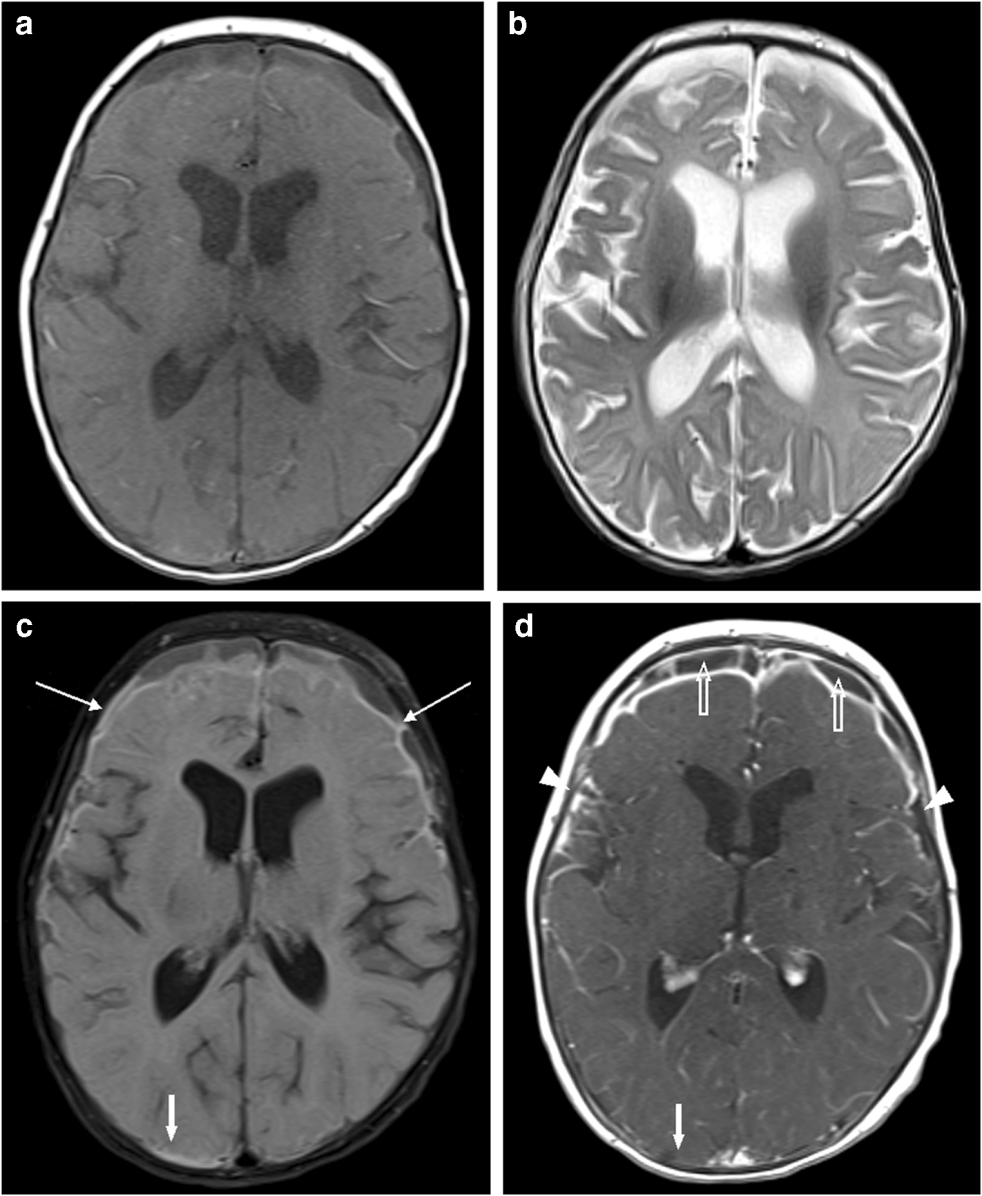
A 2-month-old girl with subdural empyema and meningitis. a–c Small subdural fluid collections in the frontal convexities bilaterally appear hypointense and hyperintense on the axial T1 (a) and T2 (b) images, respectively, with some areas of non-suppression on the axial fluid-attenuated inversion recovery (FLAIR) image (*thin arrows* in c) that suggest dural thickening and mild scalloping of the frontal cortical surface. FLAIR hyperintensity also extends slightly into the sulci suggestive of concomitant leptomeningeal disease. d The post-contrast axial T1 image shows subdural fluid collections (*open arrows*), and pachymeningeal and leptomeningeal enhancement (*arrowheads*). Note, a tiny sliver of FLAIR hyperintensity in the right occipital convexity (*thick arrow* in c) is not conspicuous on the post-contrast image (*solid arrow* in d), possibly reflecting a tiny fluid collection. Pachymeningeal and leptomeningeal enhancement seen on the post-contrast T1 image strongly suggest an infectious process and led to the imaging diagnosis of empyema and meningitis, which was later confirmed by cerebrospinal fluid analysis
